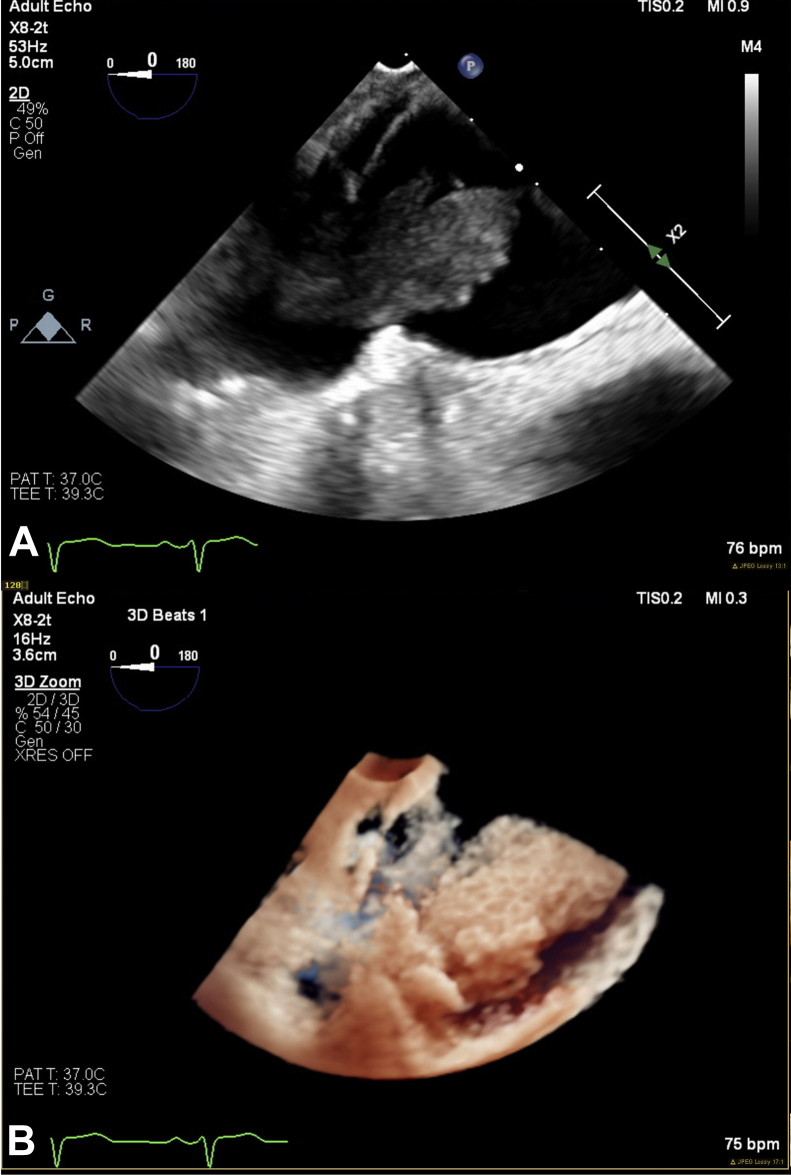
Transesophageal echocardiogram demonstrating an LA mass originating from the right lower pulmonary vein. The mass has a fixed component (39.0 × 13.3 mm) and a friable mobile segment (12.4 × 8.5 mm). Two-dimensional and associated three-dimensional TrueVue images. (A) Two-dimensional 0° view of the LA mass originating from the right lower pulmonary vein. (B) Three-dimensional TrueVue view of the LA mass from image A.
